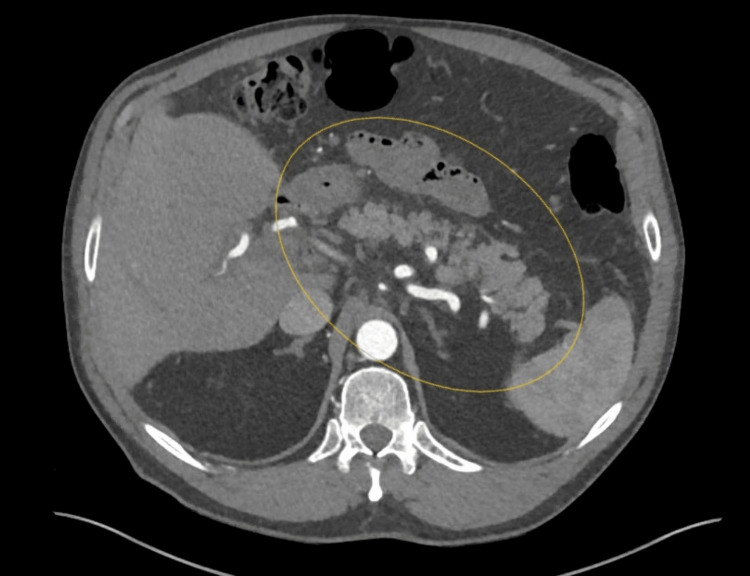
Computed tomography angiogram of the abdomen and pelvis confirms an extensive clot in the portal, splenic, and superior mesenteric veins (yellow circle).
